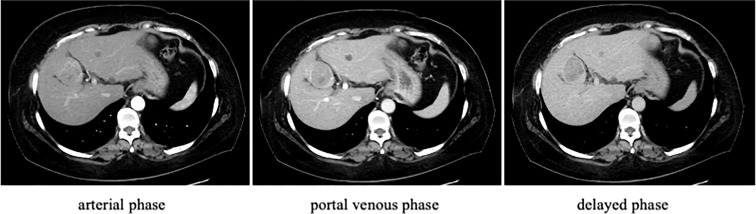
Contrast-enhanced computed tomography (CT) findings on admission. Contrast-enhanced CT of the liver showed a mass in the S4 segment of the liver that darkened in the arterial phase and washed out in the delayed phase. The liver margins were unevenly distributed.
